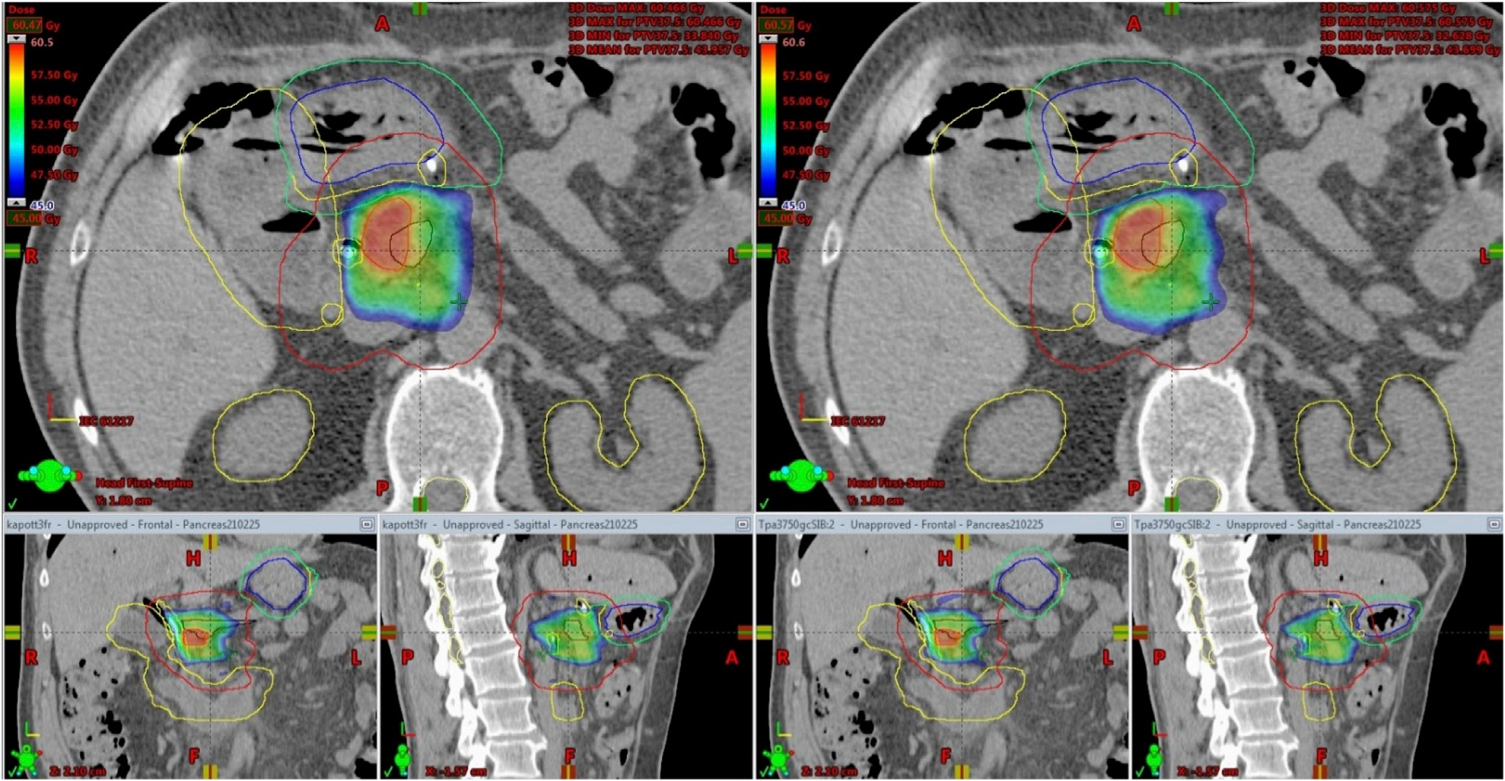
Offline treatment plan re-optimization based on CBCT contouring of the stomach (Blue: original stomach, yellow: original PRV, green: new PRV).
